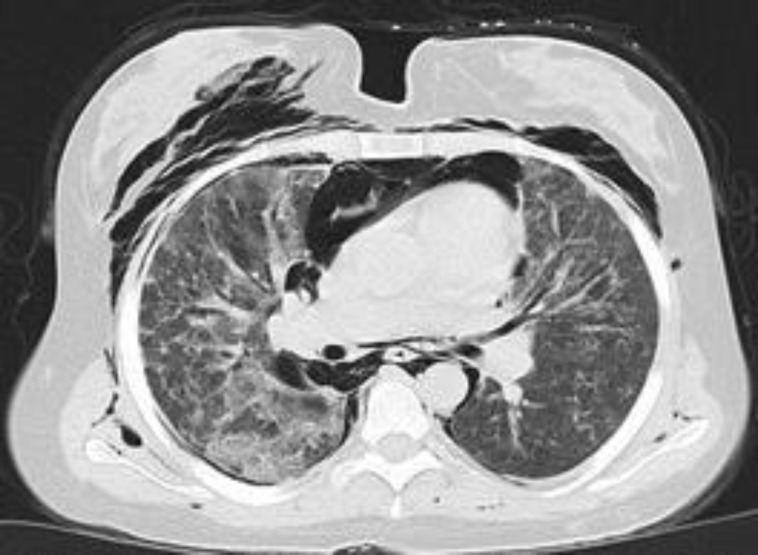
Chest computed tomography scans showing subcutaneous emphysema, pneumomediastinum, pneumothorax, thickening of interlobular septa, and a reticulonodular pattern
